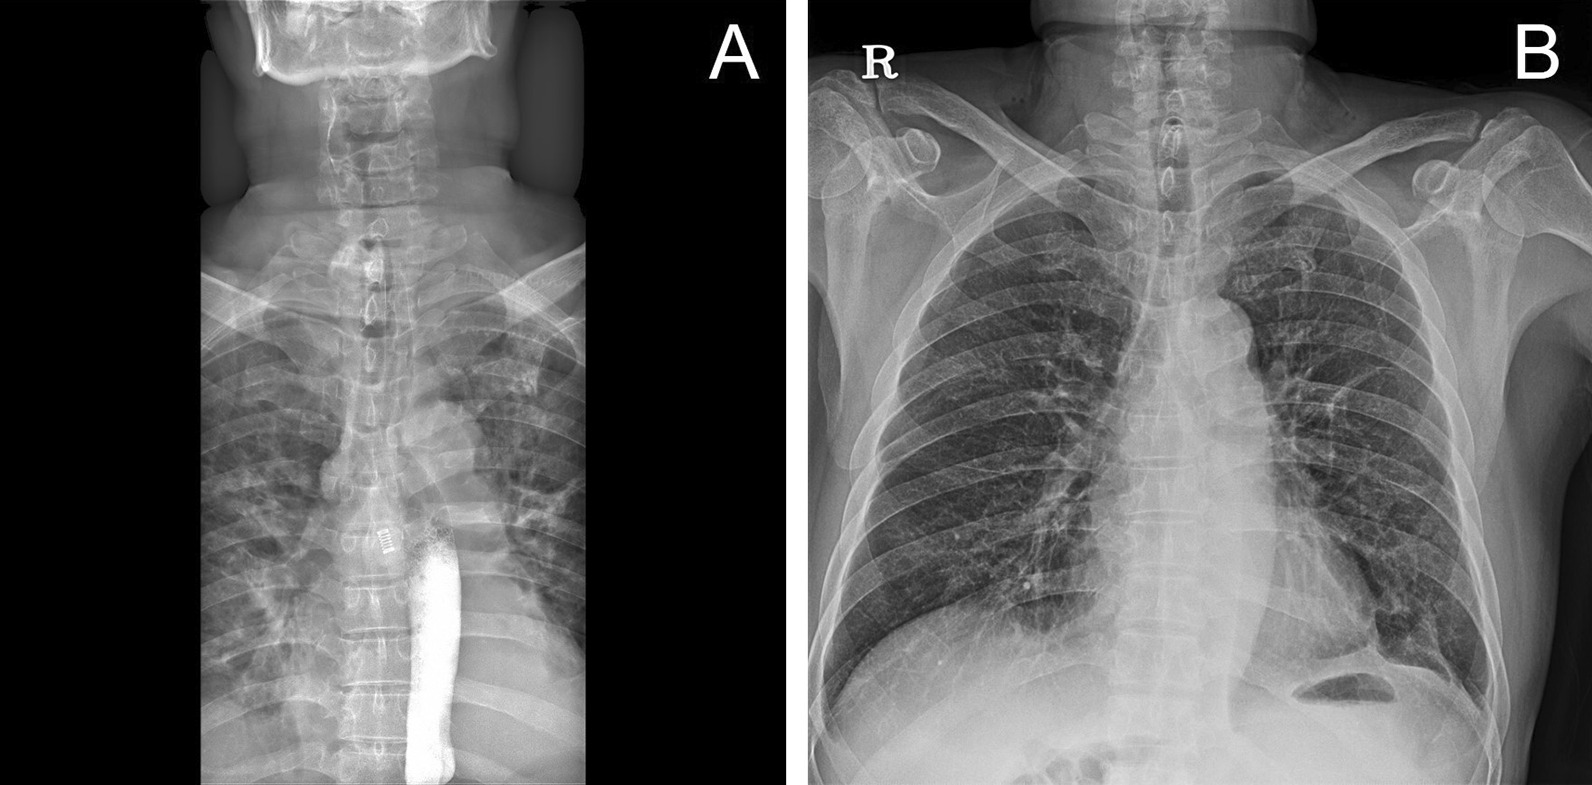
Findings at discharge. a Postoperative pharyngoesophagogram. b On the discharge day, Chest X-ray finding
